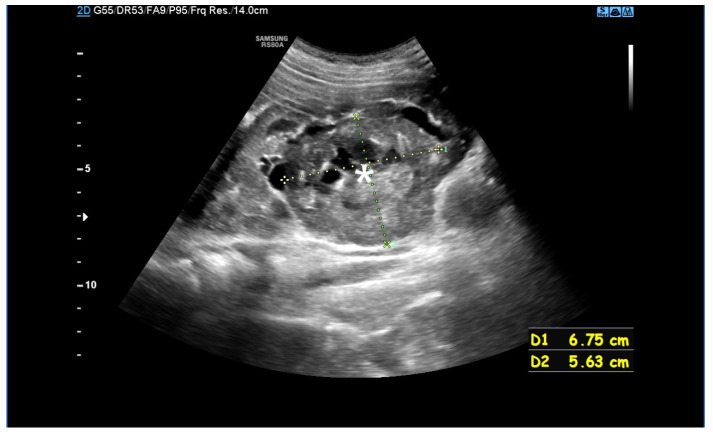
An ultrasound of a 10-year-old girl. A well-defined heterogeneous lesion, likely an AML, extending beyond the outline of the kidney. Within the lesion a fluid-filled area, likely a site of hemorrhage (*).
